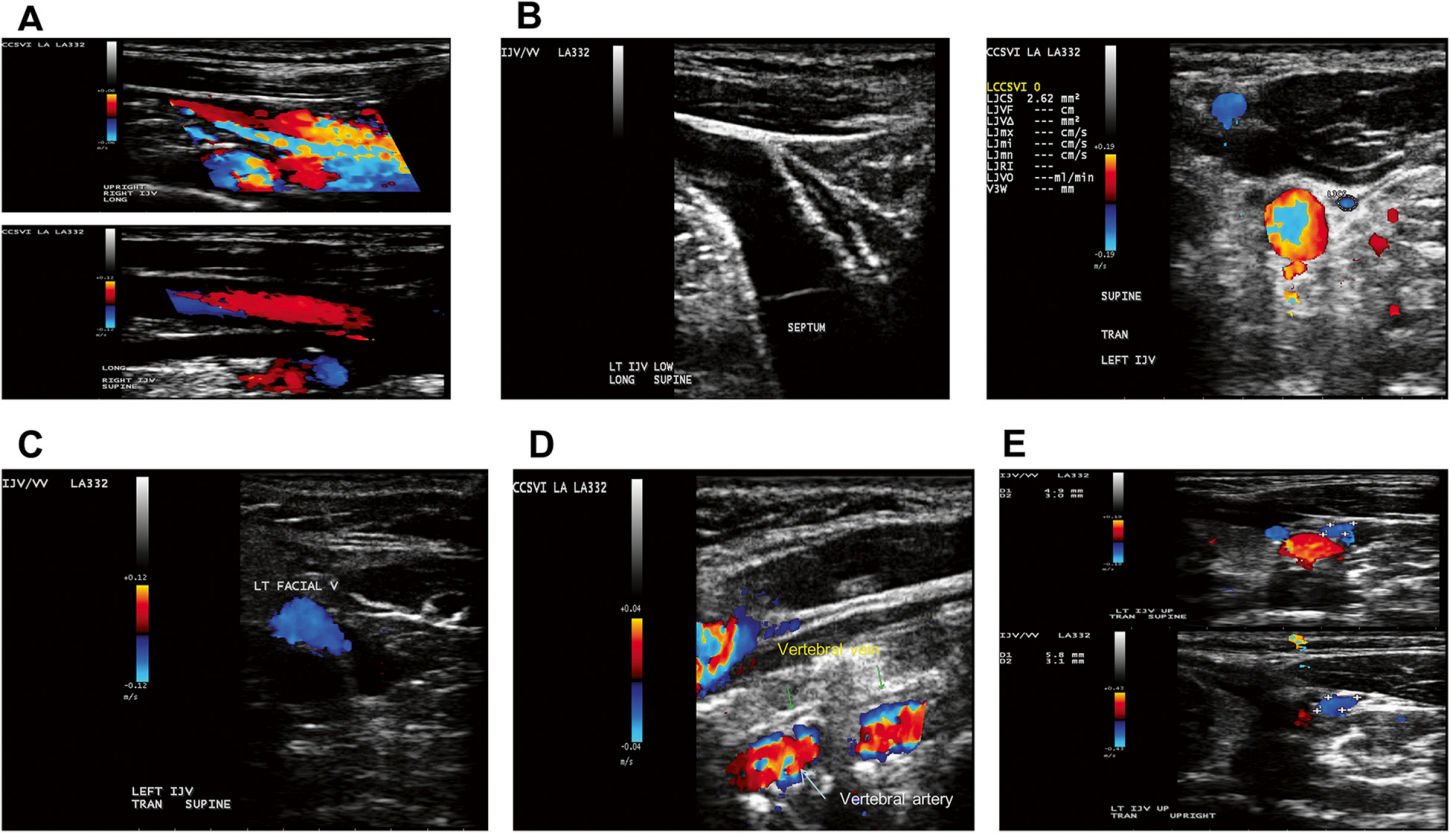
Doppler sonography venous hemodynamic (VH) criteria for diagnosis of chronic cerebrospinal venous insufficiency (CCSVI). A. VH criteria 1 - Reflux or bidirectional flow noted in the internal jugular vein (IJV) with the head in supine and upright position, B. VH criteria 3 - B-mode anomaly and/or stenosis: septum noted in the lower IJV and stenosis noted in the left IJV with a cross sectional area (CSA) ≤0.3 cm^2^; C. VH criteria 4 - No flow detected in the IJV until the facial vein enters IJV, IJV demonstrates no flow above facial vein entry; D. VH criteria 4 - No flow in vertebral veins (VV) despite low wall filter and low velocity scale; E. VH criteria 5 - Negative delta CSA – IJV cross sectional area is larger in the upright position than in the supine position resulting in a negative delta CSA (supine IJV CSA 11.54 – upright IJV CSA 12.90 = −1.36 mm).
